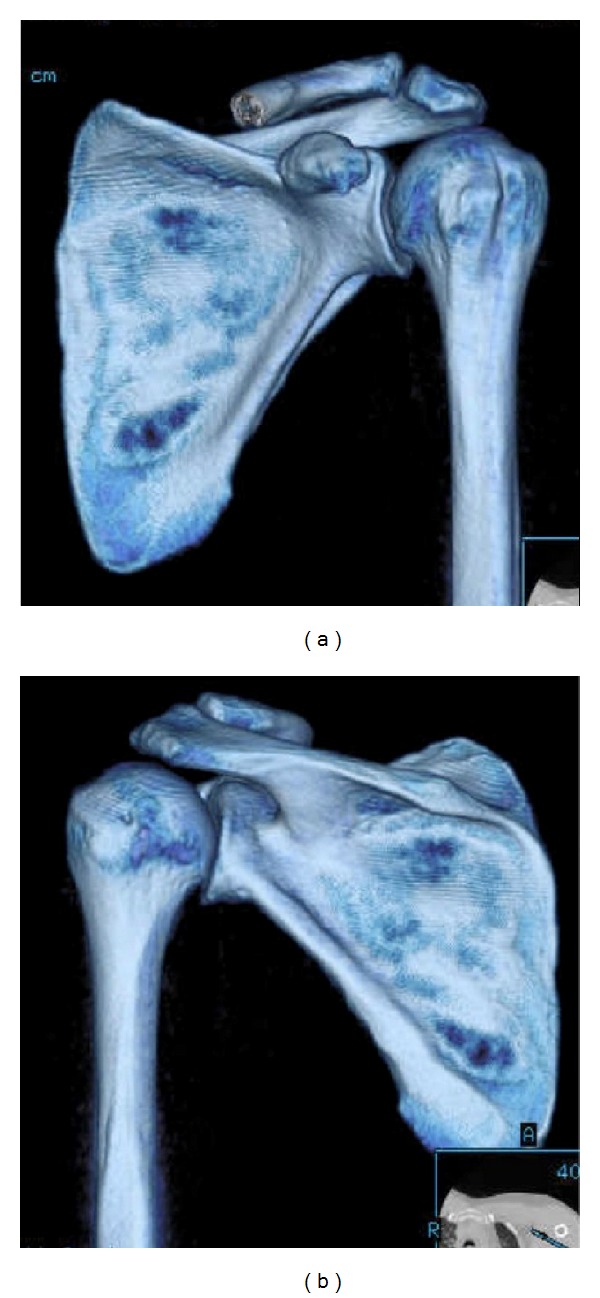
CT images with 3D reconstruction demonstrating (a) ventral surface and (b) dorsal surface of scapula.
